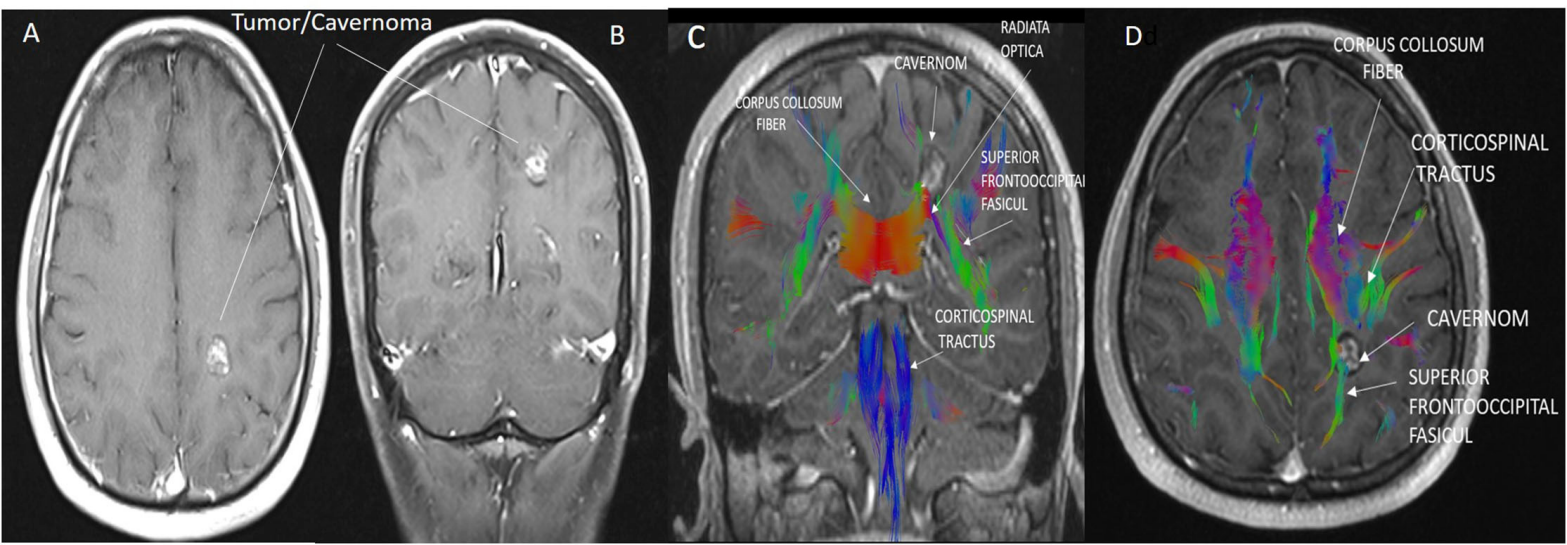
(A,B) Cavernoma appearance on axial (A) and coronal (B) contrast-enhanced T1 cranial MRI images. (C,D) The anatomical relationship of the corticospinal tract, superior fronto occipital fasciculus, and corpus callosum transverse fibers with the cavernoma is shown in sagittal and axial MRI tractography images. Due to the mass effect of the cavernoma, displacement of the superior fronto occipital fasciculus was observed.
